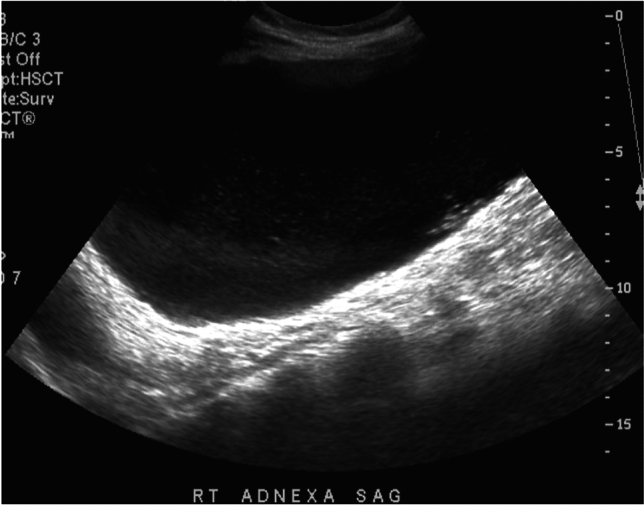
A 15-year-old female with a past medical history of epilepsy presented with hirsutism and elevated testosterone levels. Pelvic ultrasound demonstrated a large cystic structure in the right adnexum containing internal low-level echoes
